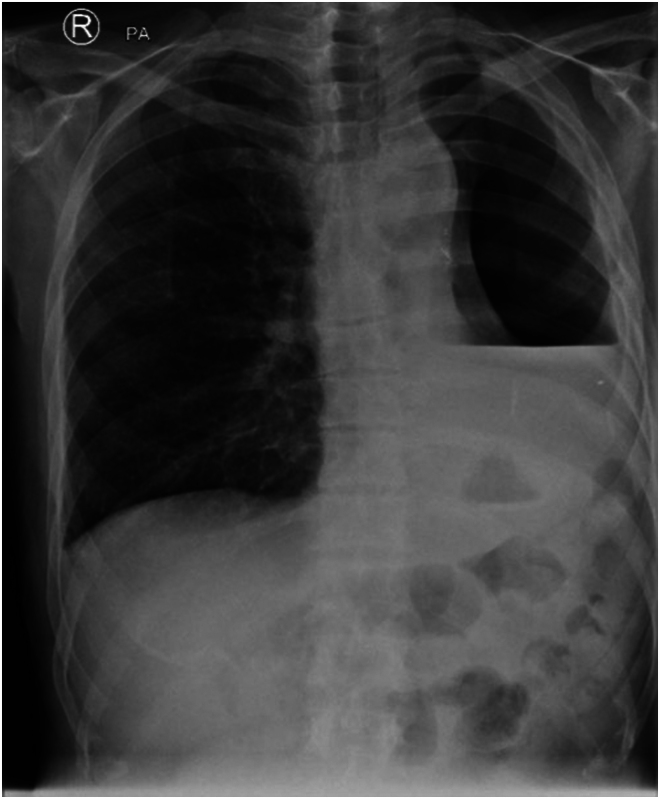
Left hydropneumothorax as identified on admission chest X-ray
